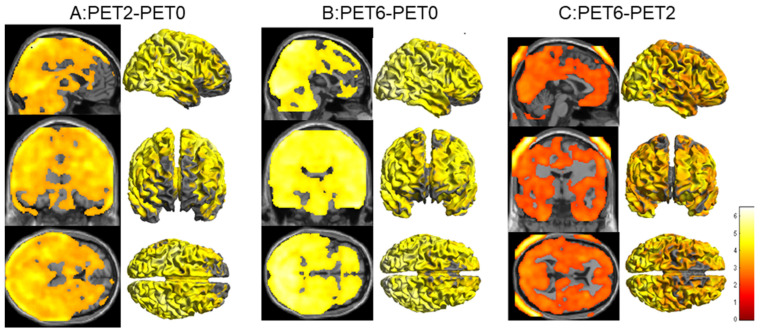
(A): Statistical parametric mapping (SPM) analysis revealed significantly increased brain metabolism in nearly the whole brain, excluding the bilateral prefrontal cortices and ventricle (overlaid in the MRI T1-weighted image and 3D cortex template), in the PET2 scan compared with that in the PET0 scan (*p* < 0.001, uncorrected). (B): SPM analysis revealed increased brain metabolism in the PET6 scan compared with that in the PET0 scan (*p* < 0.001, uncorrected). (C): SPM analysis revealed further increases in brain metabolism in the PET6 scan compared with that in the PET2 scan (*p* < 0.05, uncorrected).
